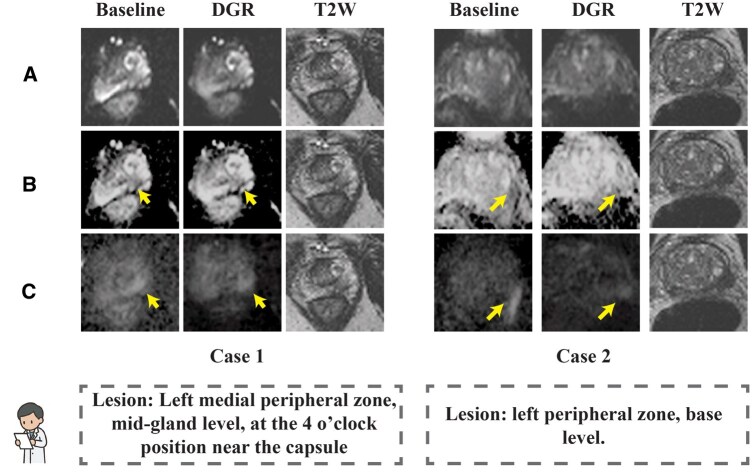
Lesion-related evaluation across 2 representative clinical cases with severe susceptibility distortion. For each case, columns show the baseline ssEPI, the proposed DGR correction, and the co-registered T_2_W reference. (A) Low-b DWI (*b* = 50 s/mm^2^), (B) ADC map, and (c) high-b DWI (*b* = 1400 s/mm^2^). Arrows indicate the tumor location on ADC and high-b DWI images. Case 1: artifact-dominated distortion with severe geometric stretching. Case 2: Lesion displacement due to extreme susceptibility-induced warping. Abbreviations: DGR, distortion-guided restoration; T2W, T_2_-weighted.
